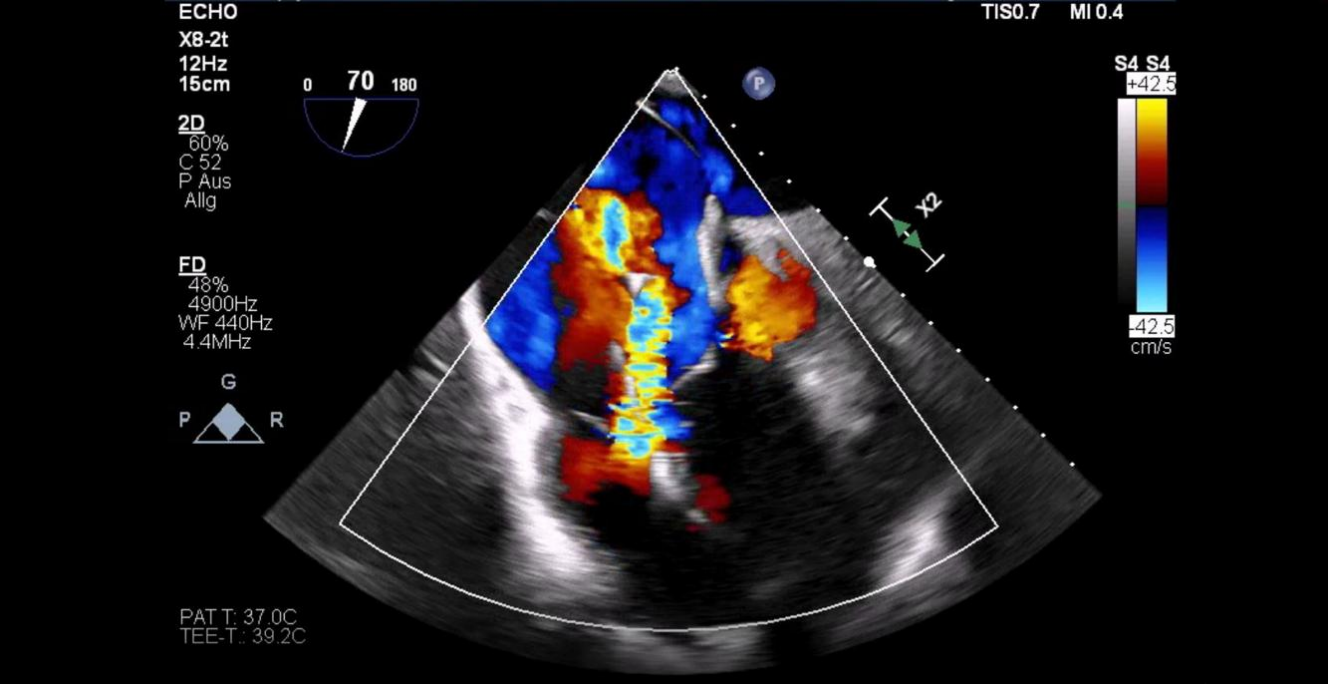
Transoesophageal echocardiography demonstrating severe tricuspid regurgitation prior to EVOQUE™ implantation. The regurgitant jet is aligned with the intracardiac device lead.
